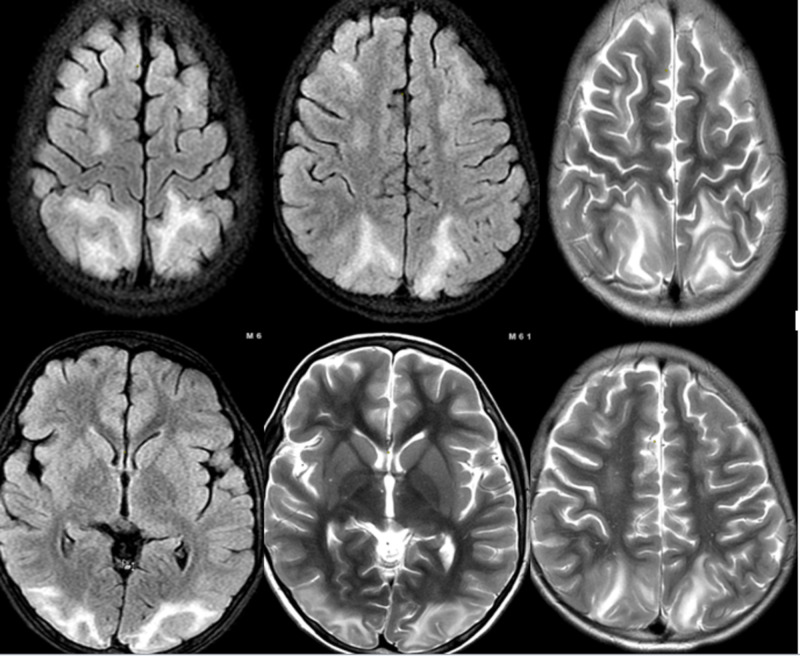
T2 FLAIR (fluid attenuated inversion recovery imaging) image showing the involvement of subcortical and deep white matter of bilateral parieto-occipital lobes, right temporal lobe and bilateral frontal lobe
